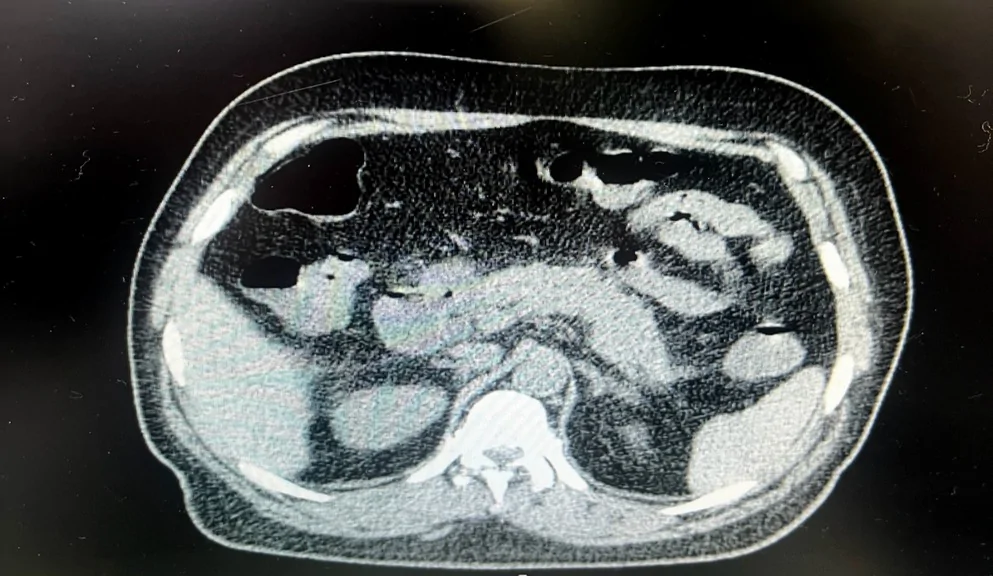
Abdomen Computed Tomography (CT) Scan; Pancreatic swelling and surrounding fat stranding.
